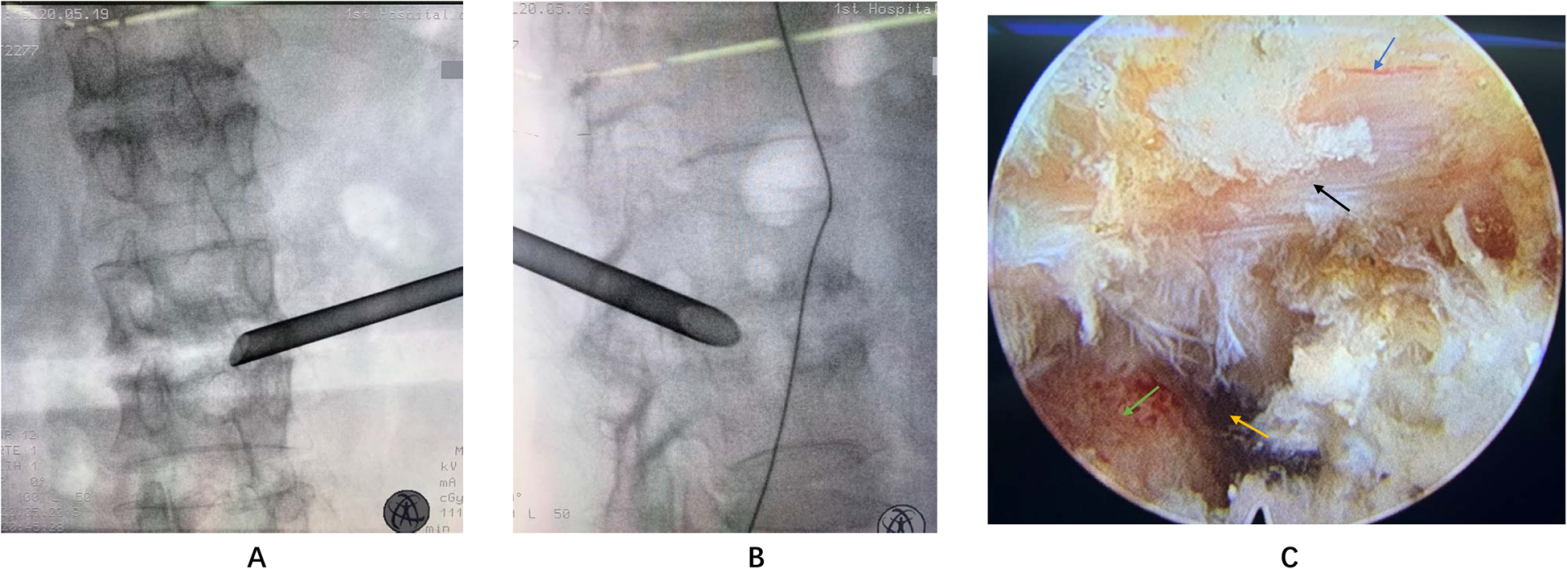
Surgical process a lumbar anteroposterior X-ray film. b lumbar lateral X-ray film. c view of PTED (blue arrow: nerve root; black arrow: ligamentum flavum; green arrow:end plate; yellow arrow: intervertebral space)
